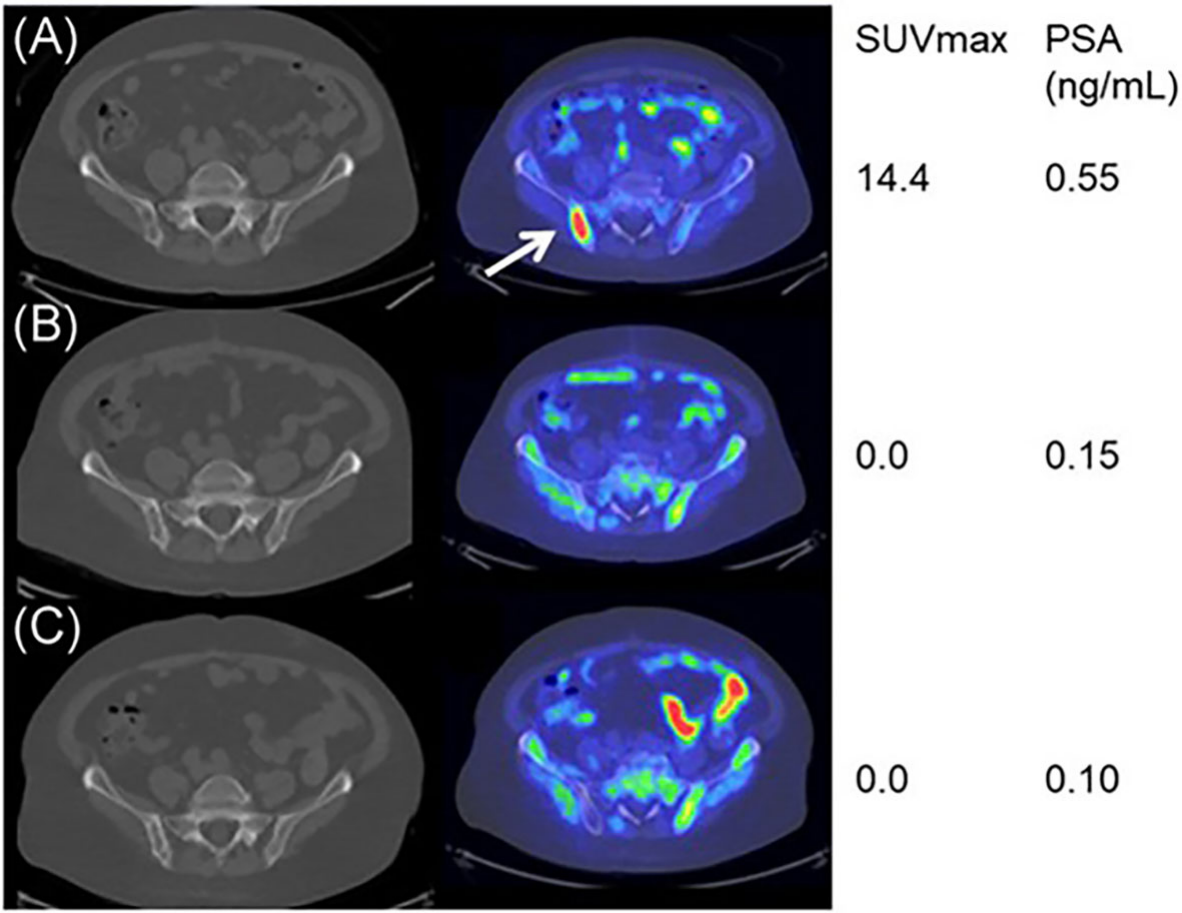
^11^C‐choline PET/CT imaging during the docetaxel chemotherapy for a good responder. Baseline (A), mid‐course (B), and posttherapy (C) axial fused ^11^C‐choline PET/CT images demonstrating markedly choline‐avid right posterior iliac bone metastasis at baseline (arrow), while nearly none at mid-course and posttherapy. Reprinted with permission from Jimbo et al. (54). Copyright ^©^2021 Wiley Periodicals LLC.
